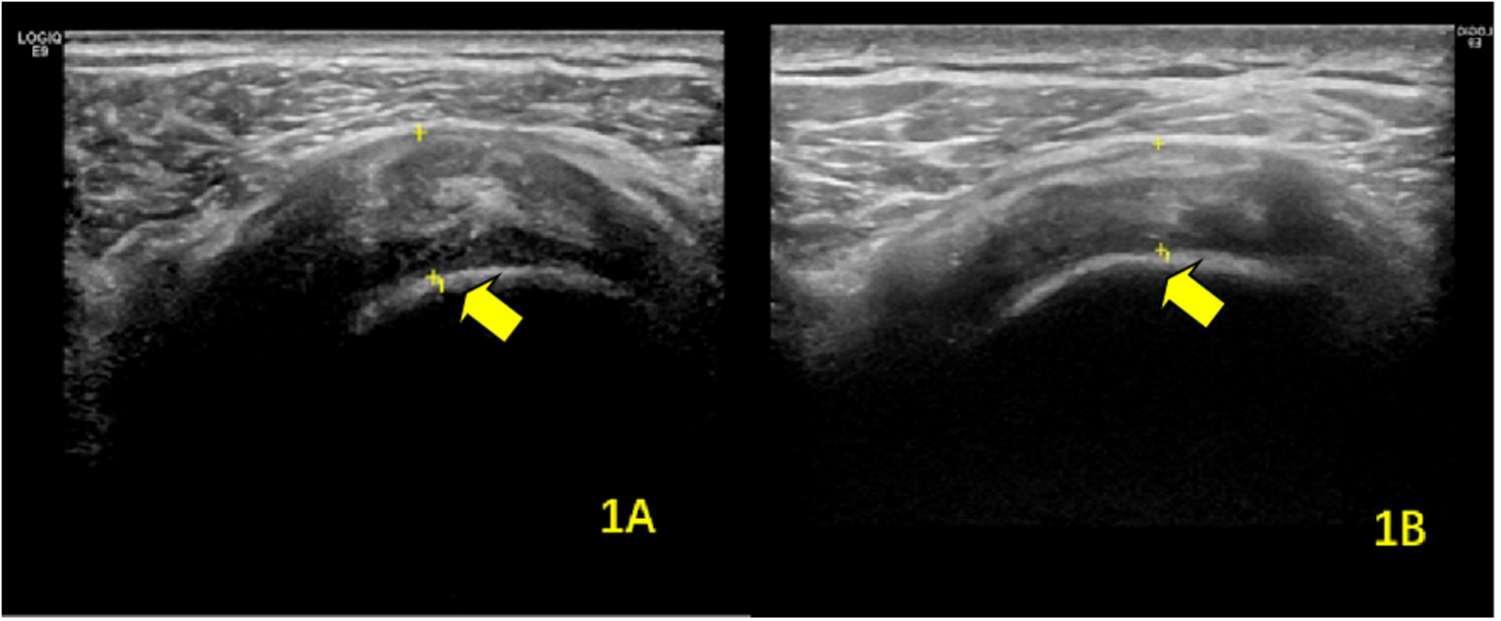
Ultrasonographic findings of supraspinatus tendinitis in the hemiplegic shoulder (A) before and (B) after rehabilitation therapy. The arrows indicate the affected supraspinatus tendon.
